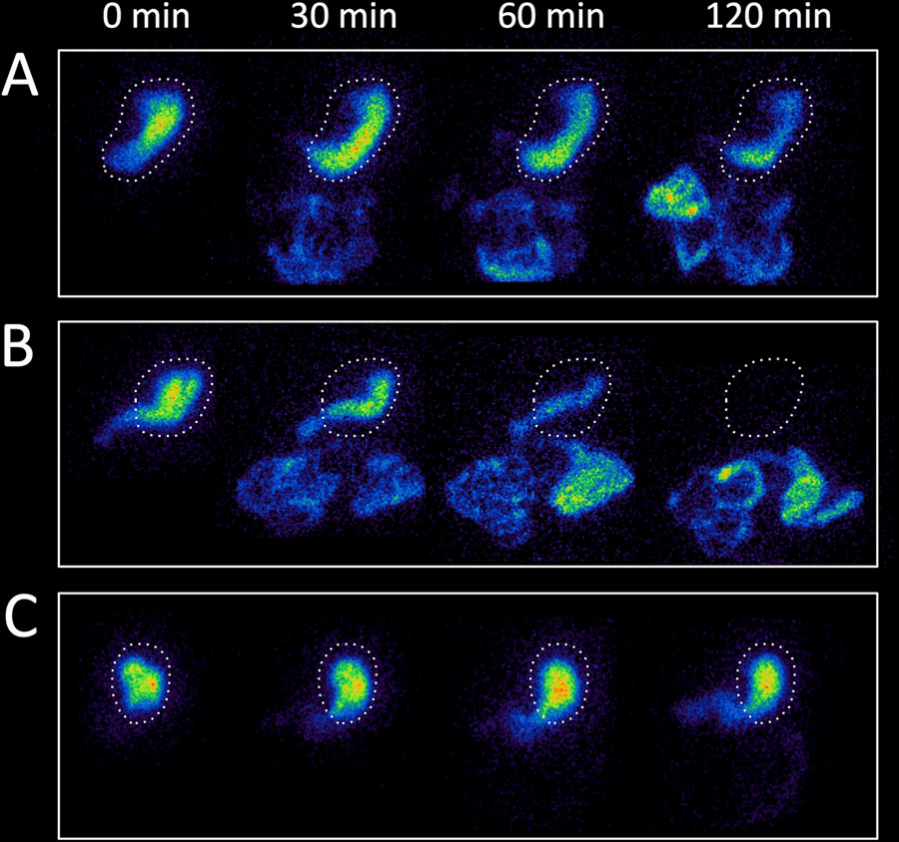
Gastric emptying scintigraphy images at 0, 30, 60, and 120 min after radioactive meal ingestion. a Healthy control with normal gastric emptying time (*T*
